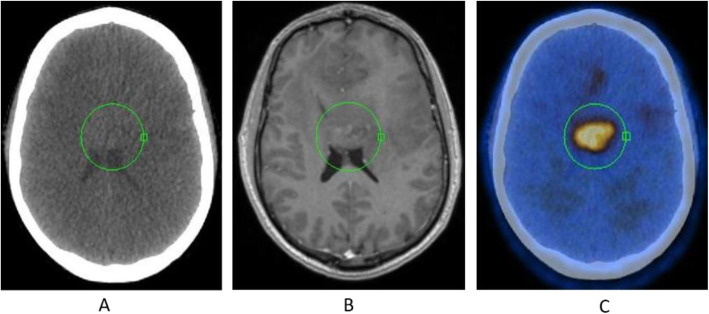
^18^F-FET, evaluation of a brain lesion. *Clinical history*: 46 y.o. man with nausea and headache. CT was non-informative (a). MRI shows an infiltrating lesion with low Gd enhancement (b). *PET/CT findings*: high FET uptake (c). Surgery confirmed a high-grade glioma
